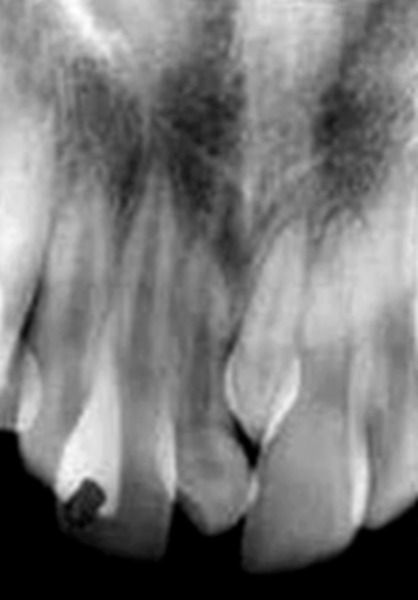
Periapical radiograph showing absence of unerupted supernumerary teeth
